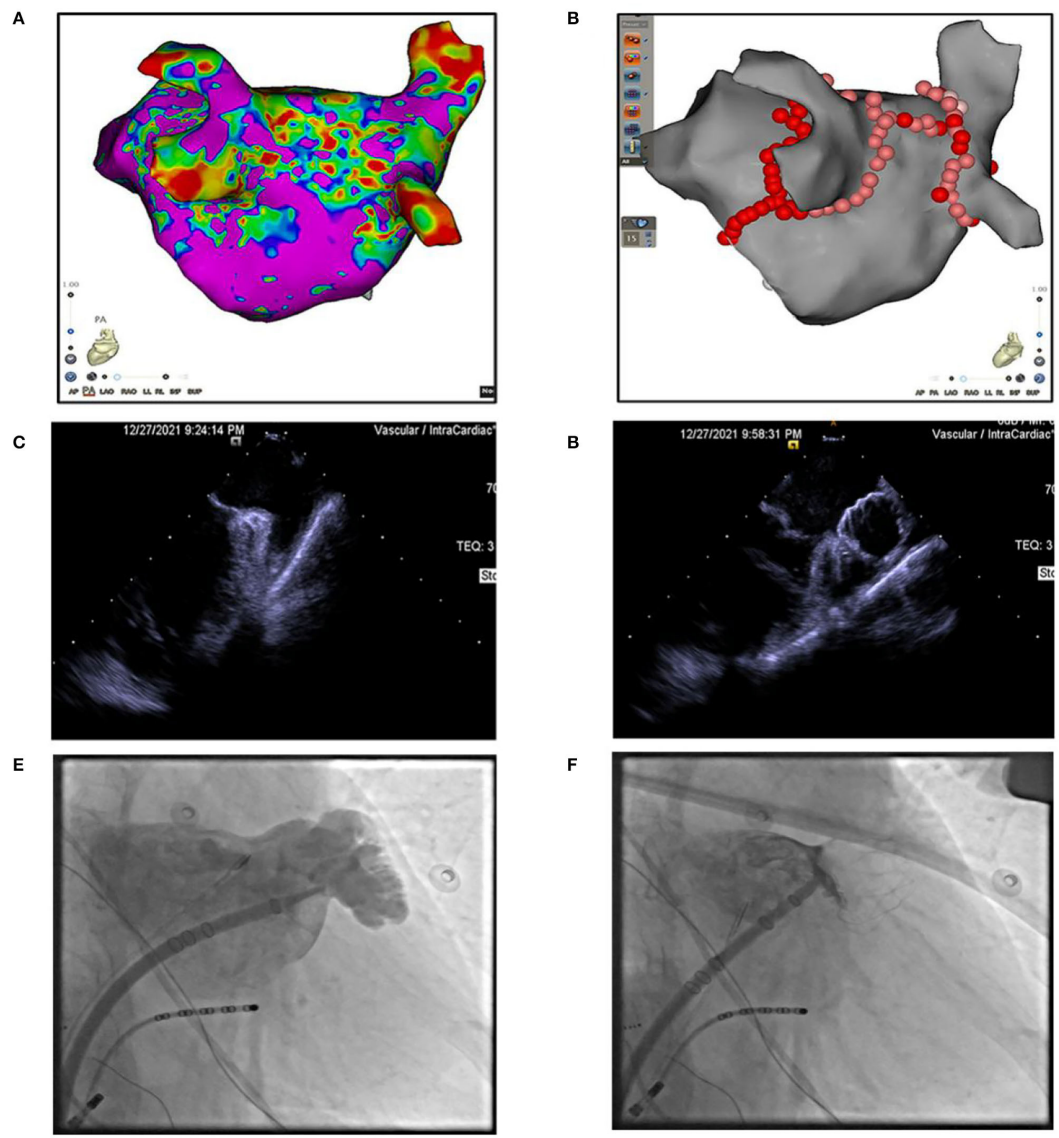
(A) 3D modeling and substrate mapping of left atrium. (B) Catheter ablation at pulmonary veins, left atrium roof, and mitral isthmus. (C,D) Intracardiac echocardiography image of left atrial appendage (LAA) before or after LAA occlusion. (E,F) Fluoroscopy image of left atrial appendage (LAA) before or after LAA occlusion.
